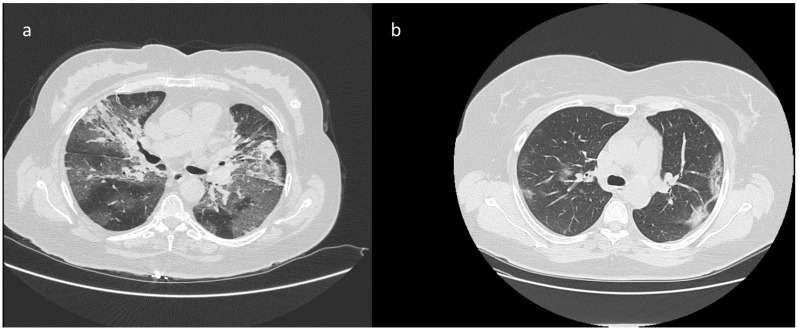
Patients with a positive RT-PCR test for COVID-19 infection. The CT exams detected areas of GGO and parenchymal consolidation with multifocal diffuse (a) or predominantly peripheral distribution (b).
